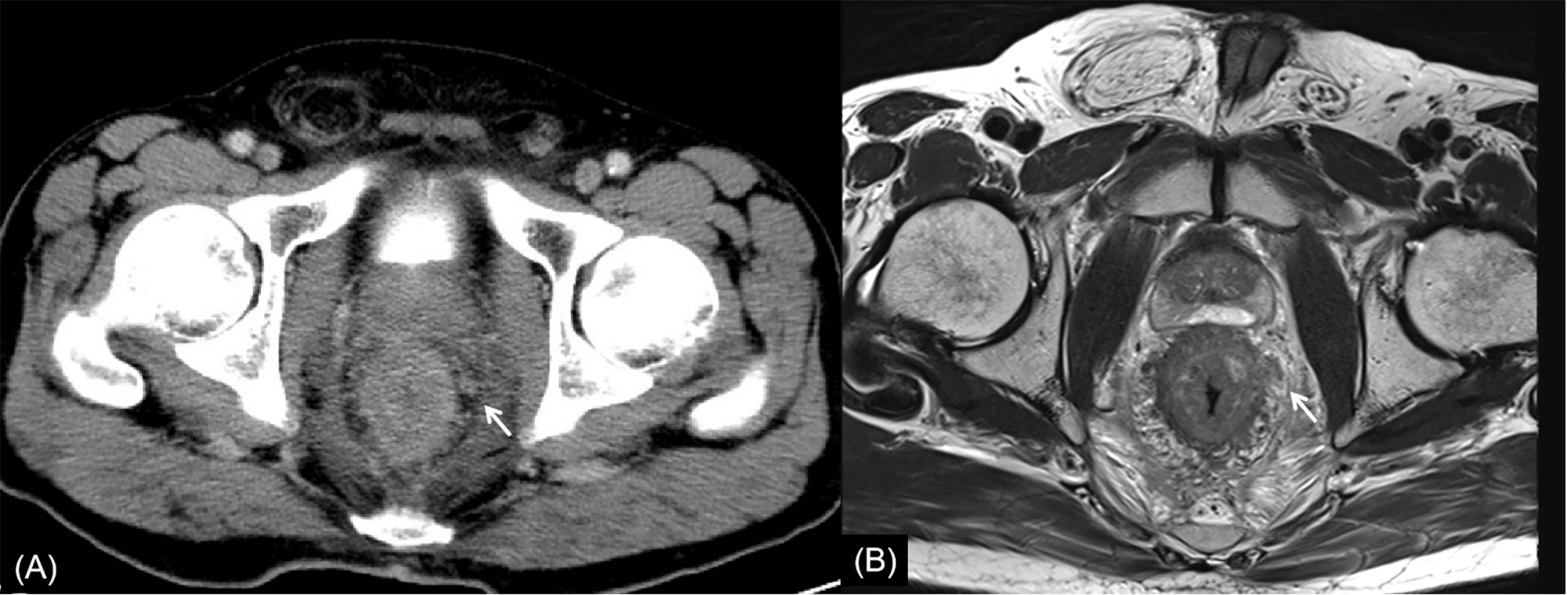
Rectal cancer with ypT4N2. (A) Axial MSCT depicts whole rectal wall thickening with uneven density of adjacent mesorectum. Low restaged as T3. (B) MRI transverse high-resolution T2WI shows thickened whole rectal wall with extensive edema and fibrosis of mesorectum. Low restaged as T3. Enlarged lymph node (arrow) was found. Accurately restaged as N2 with MSCT and MRI. yp: pathological staging after neoadjuvant chemoradiotherapy; T, tumor; N, node; MSCT, multi-slice computed tomography; MRI, magnetic resonance imaging.
